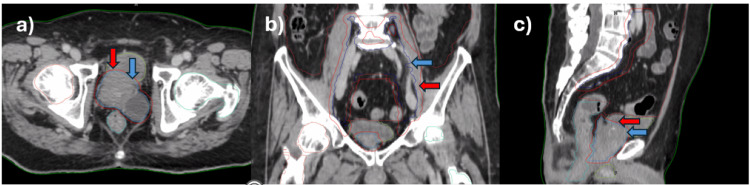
Planning CT images showing target and OAR contours in (a) axial, (b) coronal, and (c) sagittal sections. In pane a, the blue contour is CTVp (blue arrow), and the red contour is PTVp (red arrow). In pane b, the blue contour is CTVn (blue arrow), and the red contour is PTVn (red arrow). In pane c, CTVp (blue arrow) and PTVp are visualized CT: computed tomography, OAR: organs at risks, CTVp: clinical target volume of the primary tumor, PTVp: primary planning target volume, CTVn: clinical target volume of nodes, PTVn: planning target volume nodes
